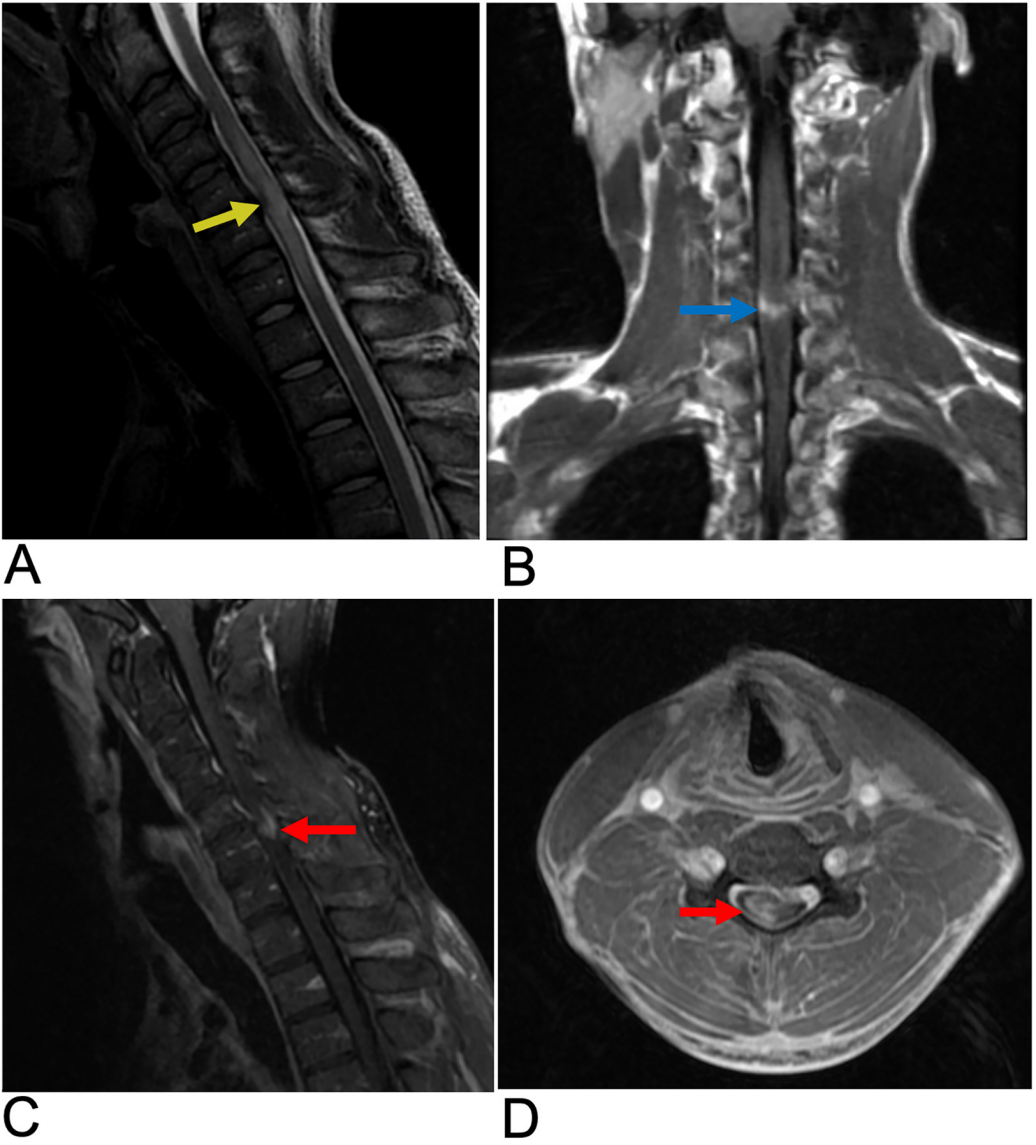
Spinal cord injury. Sagittal T2 PROP (A), coronal T1 FLAIR + C (B), sagittal T1 FLAIR FAT + C (C), and axial 3D FSPGR Fat + C (D) MRI of the cervical spine showing C5-C6 cord compression (yellow arrow) resulting from posterior disc/osteophyte complex herniation. There is associated enhancement of the right hemi-cord (blue arrow) in the “pancake configuration” (red arrows) representing compressive myelopathy
